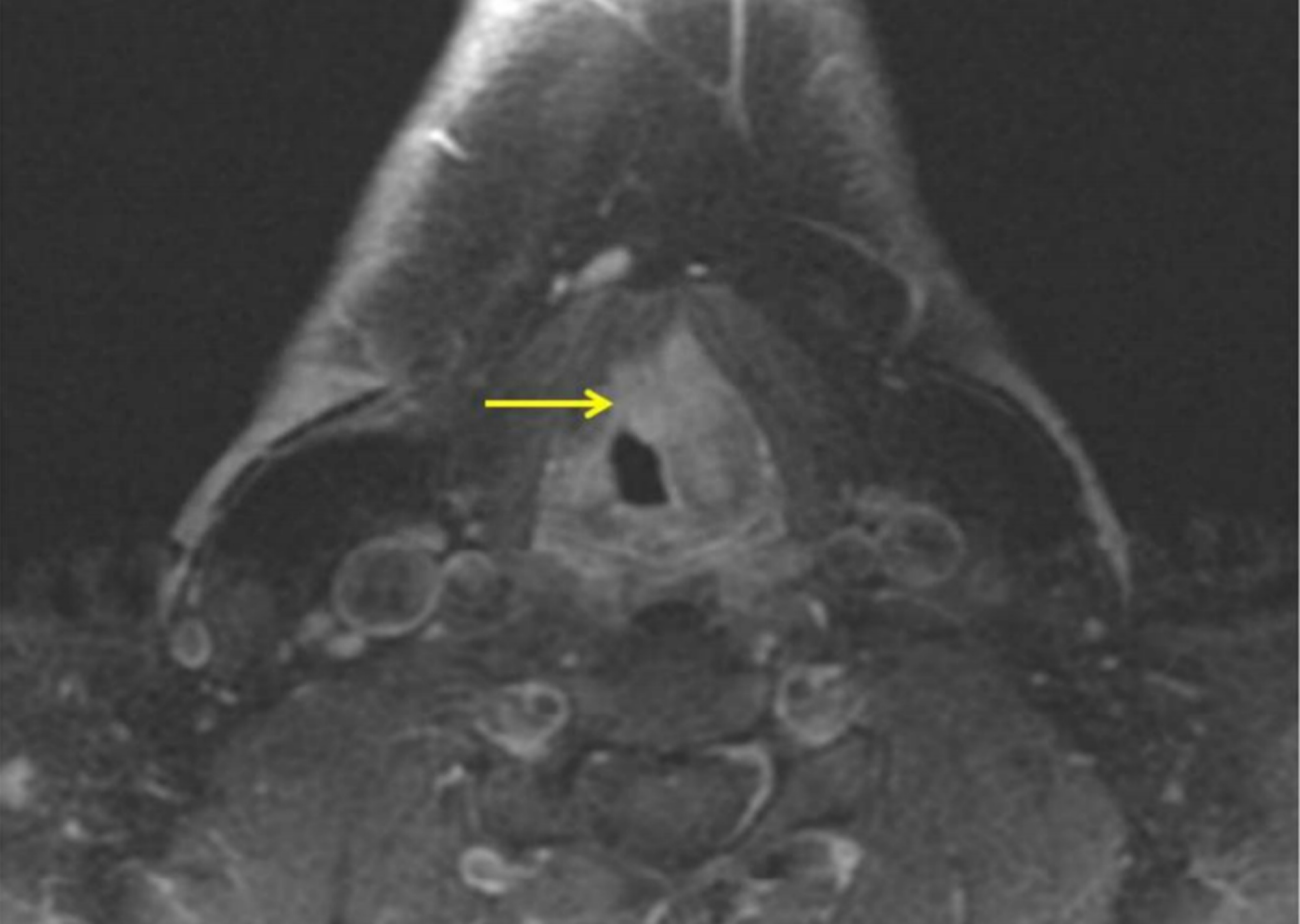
Axial T1 post-contrast magnetic resonance imaging (MRI) Ill-defined enhancing soft tissue mass (arrow) centered in the left false vocal cord and paraglottic fat, extending to the left true vocal cord, anterior commissure, and left aryepiglottic fold.
